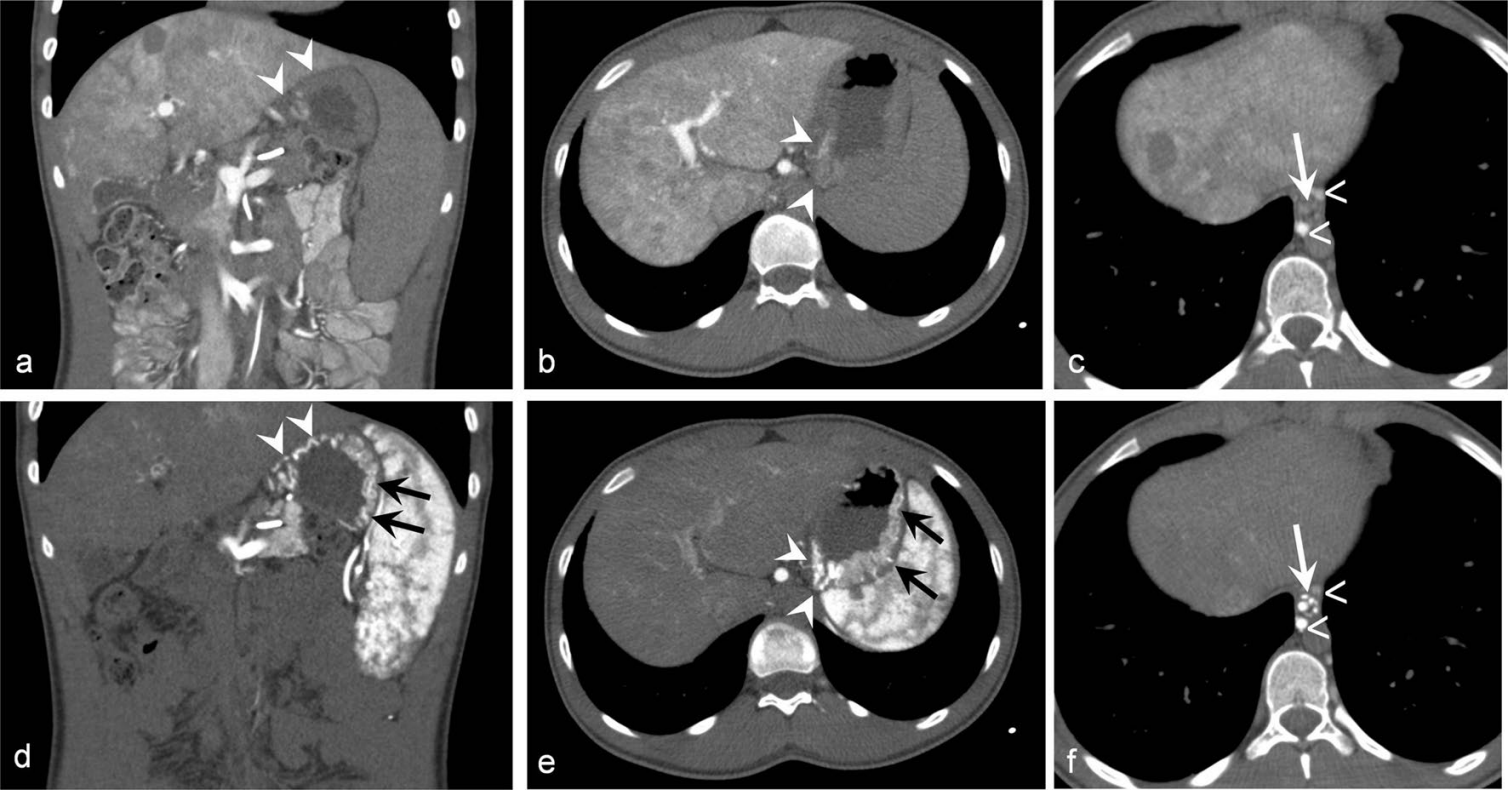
A 15-year-old male patient with PH. Coronal and axial images of CT-AP (a–c) and CT-AS (d–f). Enhancement of varices of the gastric cardia (arrowheads) and esophagus (white arrows) predmominates in CT-AS (d–f) in comparison to CT-AP (a–c). Enhancement of paraesophageal varices (open arrows) in both CT-AP (c) and CT-AS (f). In UEG varices at the gastric cardia were described, CT-AS (d, e) delineates additional small fundic varices (black arrows).
